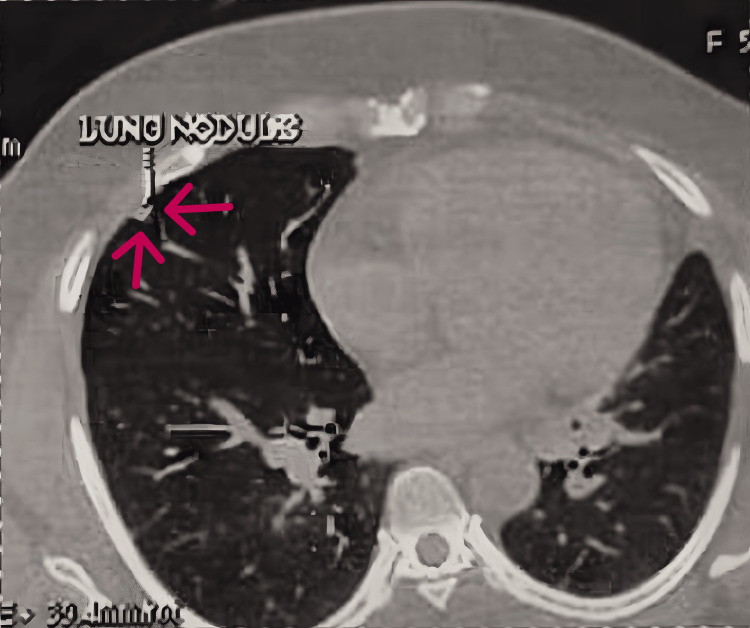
Axial section of the computed tomography of the thorax showing a subpleural nodule (indicated by red arrows) in the right upper lobe
